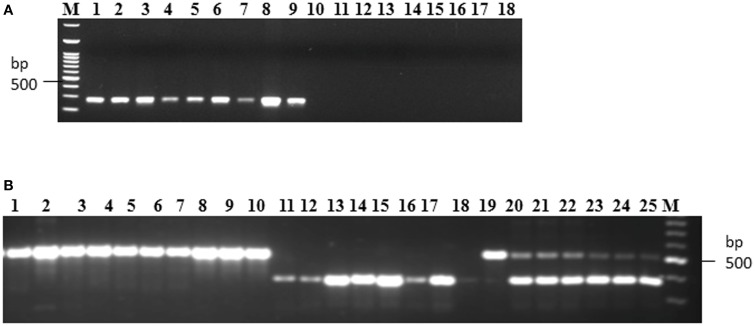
PCR amplification of rice lines with the dominant and co-dominant PCR marker. (A) PCR amplification with the dominant marker (D163_F and D163_R) in 18 rice lines. Lanes 1–9: presence of band in rice lines (Acharmati, Aditya, Ananda, Beesginsali, Bidarlocal-2, Dhanaprasad, Gautam, Himdhan, and Pusa sugandh 5); lanes 10–18: absence of band in rice lines.(HR-12, TP-309, Co-39, Nipponbare, Shiva, Satti, Pusa basmati-1, Ram Jawain-100, and Sathia-2) (B) PCR amplification with the co-dominant marker (C144_F and C144_R) in 26 rice lines. bp- base pair; M- 100 bp molecular marker. Lanes 1–10: presence of susceptible band (CO-39, HR-12, CT-10006-7-2M-5-1P3M, Jyoti, Kavali kannu, Mesebatta, Nipponbare, Samba mahsuri, Shiva, Taipei-309); lanes 11–18: presence of resistant band (Tetep, Budda, Chiti zhini, CSR 10, Gonrra bhog, Himalya 799, Pusa Sugandh 3, Ranbir basmati, Sadabahar); lanes 19–25: presence of both the bands (Belgaum basmati, CSR-60, HLR-142, Indrayani, IRAT-144, Kariya).
